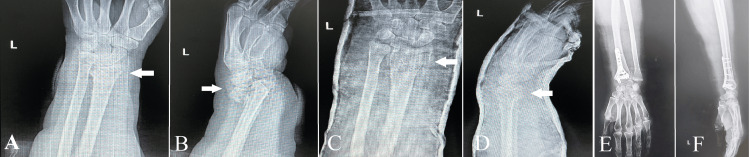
Case 1 - arrows point to the comminuted fracture This image displays the radiographs of a patient upon their arrival in the emergency room, revealing a severely fragmented fracture at the distal radial epiphysis (images A and B). An orthopedic reduction procedure was carried out, resulting in a satisfactory alignment considering the complexity of the fracture (as shown in images C and D). Due to the patient's advanced age and the low quality of their bones, osteosynthesis was performed to stabilize the fracture (images E and F), showcasing a strategic approach to handling difficult cases like this.
